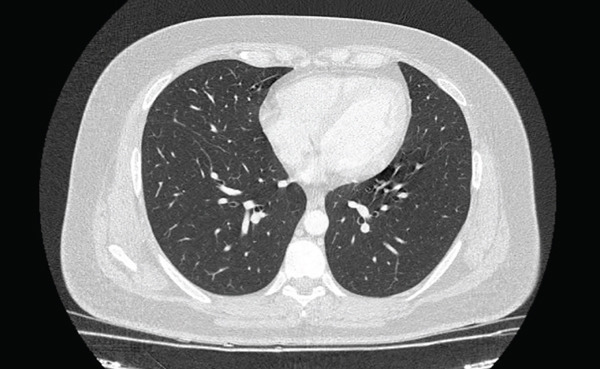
A lung computed tomography scan demonstrated cystic bronchiectasis in the lingula and linear atelectasis in the left lung.
